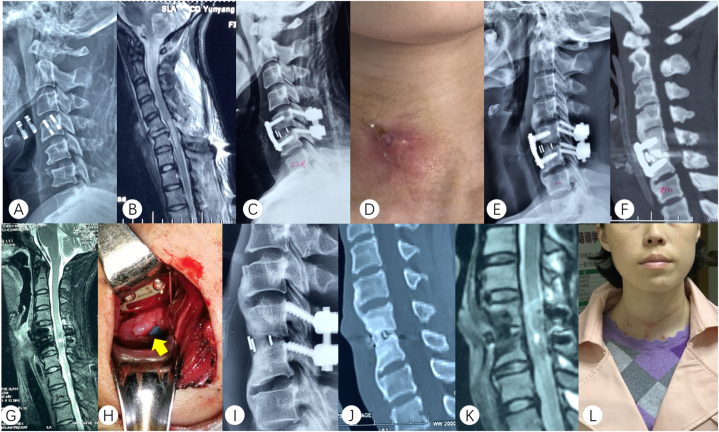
A 32-year-old female presented with a cervical fracture and cervical spinal cord injury. Preoperative DR (A) Preoperative MRI (B). DR after internal fixation treatment (C). Photograph of the neck incision 8 months post-surgery (D). DR at 8 months post-surgery (E). CT at 8 months post-surgery (F). MRI at 8 months post-surgery (G). Intraoperative photos of the second operation (H). DR at 4 months after the second operation (I). CT at 4 months after the second operation (I). MRI at 4 months after the second operation (I). General picture of the patient at 4 months after the second operation (L).
